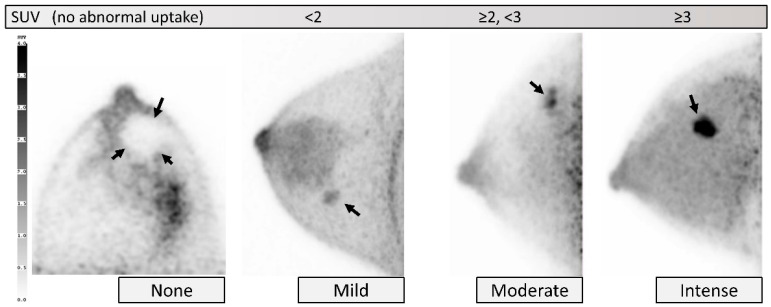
Lesion: Intensity. From left to right, *none* (cyst; tomographic image), *mild* (category 2 on ultrasound; MIP), *moderate* (intraductal papilloma; MIP), and *intense* (invasive ductal carcinoma; MIP). Intensity is classified using SUV scale as a guide. Arrows indicate target lesions.
